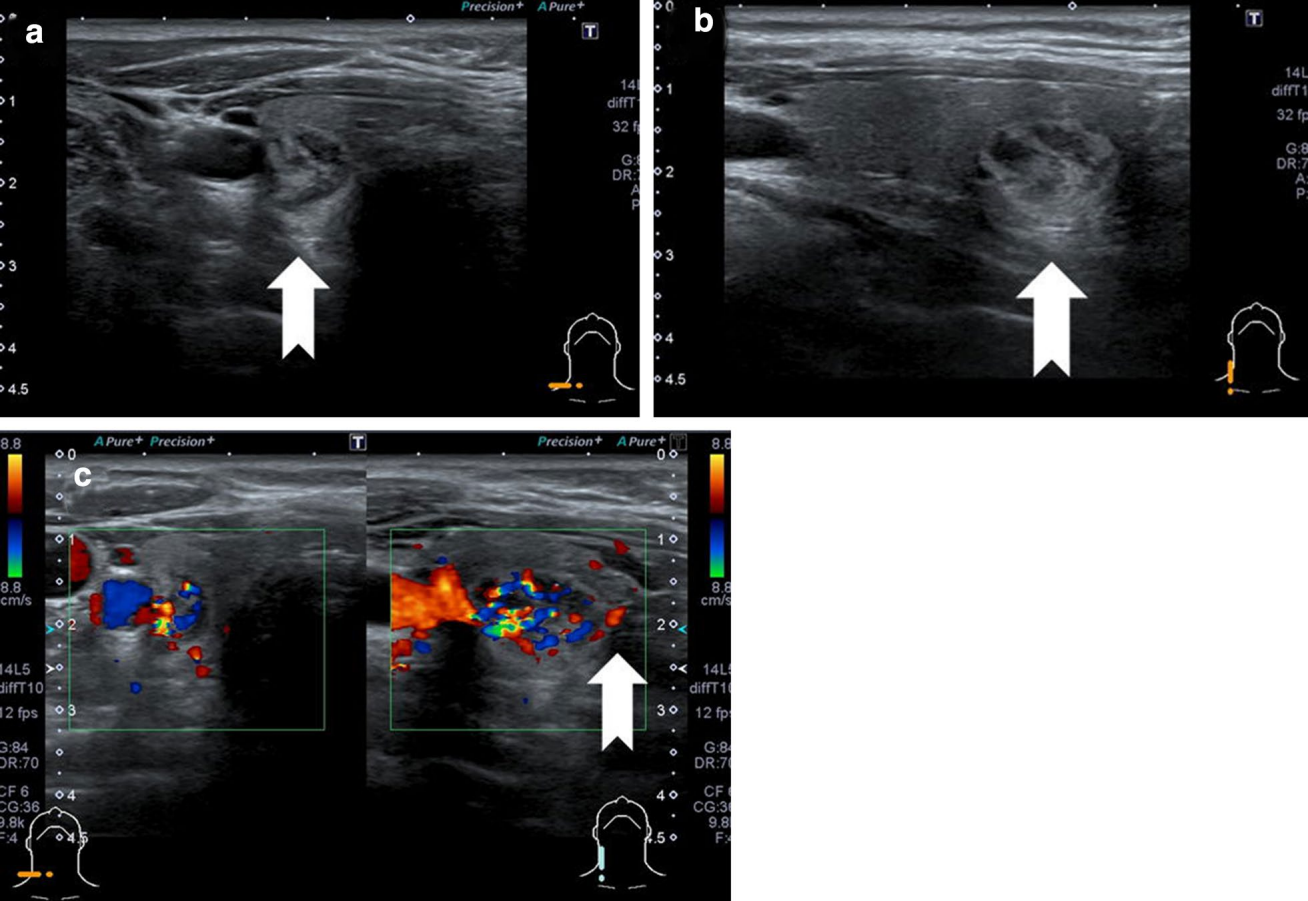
Ultrasonographic imaging. a Transverse image. b Longitudinal image. c Color Doppler imaging. The tumor ((a, b) arrow) was located in the inferior pole of the right lobe of the thyroid gland and no parathyroid mass was observed. The size of the tumor was 18 × 13 × 11 mm (anteroposterior × transverse × craniocaudal dimension). The tumor had an irregular round shape and showed heterogeneous hyperechogenicity with a defined margin, but within it, there were a few unclear margins, irregular and hypoechogenic area, and the tumor had a mosaic appearance at first glance. Color Doppler sonography showed that the blood vessels ((c) arrow) flowing into the tumor were thick and marked blood flow was also observed within the tumor
